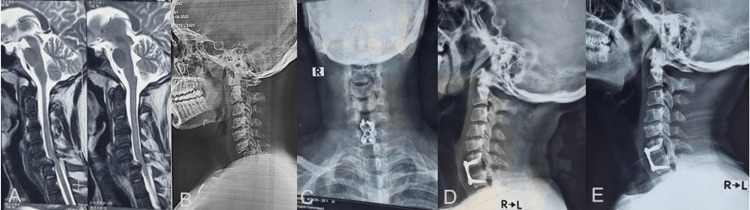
Anterior cervical discectomy and fusion (ACDF) A. Preoperative T2-weighted sagittal section of the cervical spine B. Preoperative X-ray of the cervical spine in lateral view C: Postoperative X-ray of the cervical spine in the antero-posterior and (D) lateral view E: X-ray of the cervical spine in lateral view at final follow-up (fusion)
